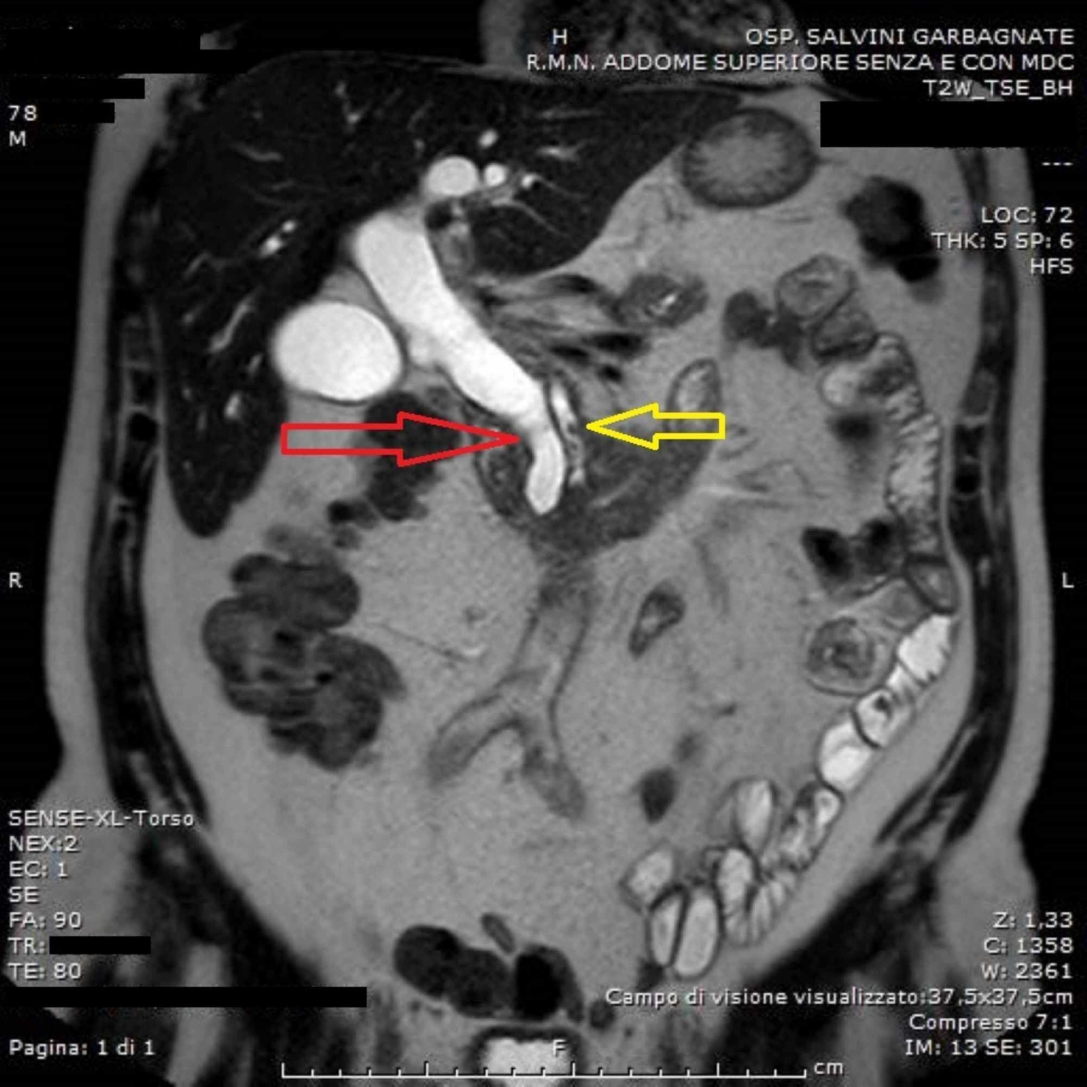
Abdominal magnetic resonance imaging (MRI): dilatation of the common bile duct (red arrow) and the major pancreatic duct (yellow arrow).
